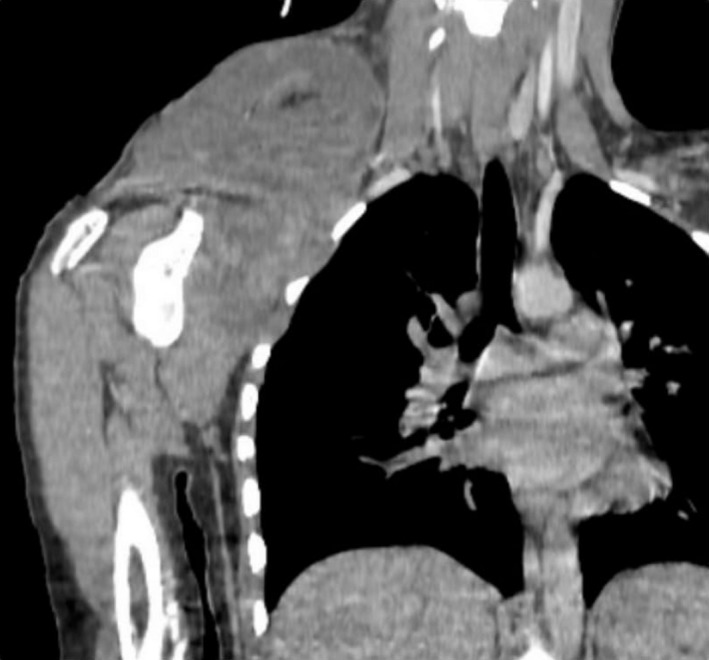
CT scan showing a voluminous tumor of the right supraclavicular region extending over 9 cm to the 5th intercostal space
